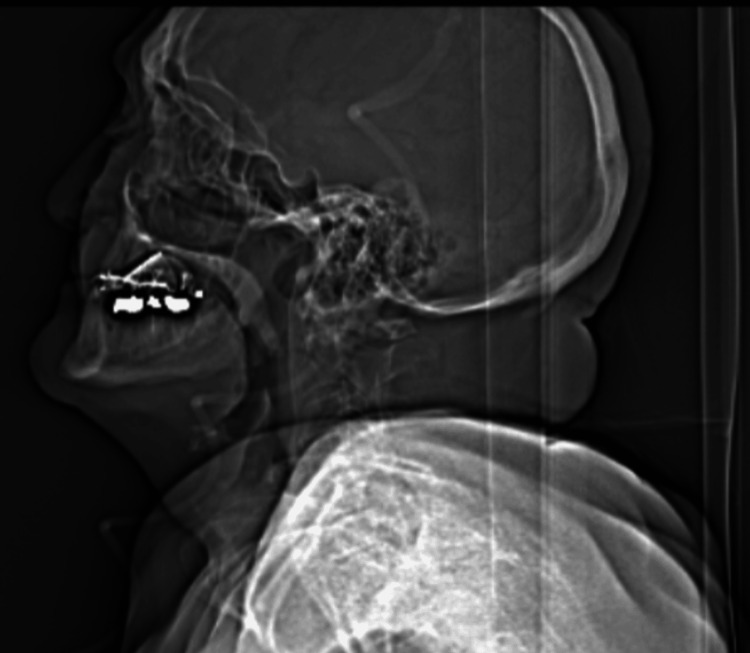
CT scan of the neck demonstrating no discernible mass or focal abnormality within the larynx or elsewhere within the upper aerodigestive tract
